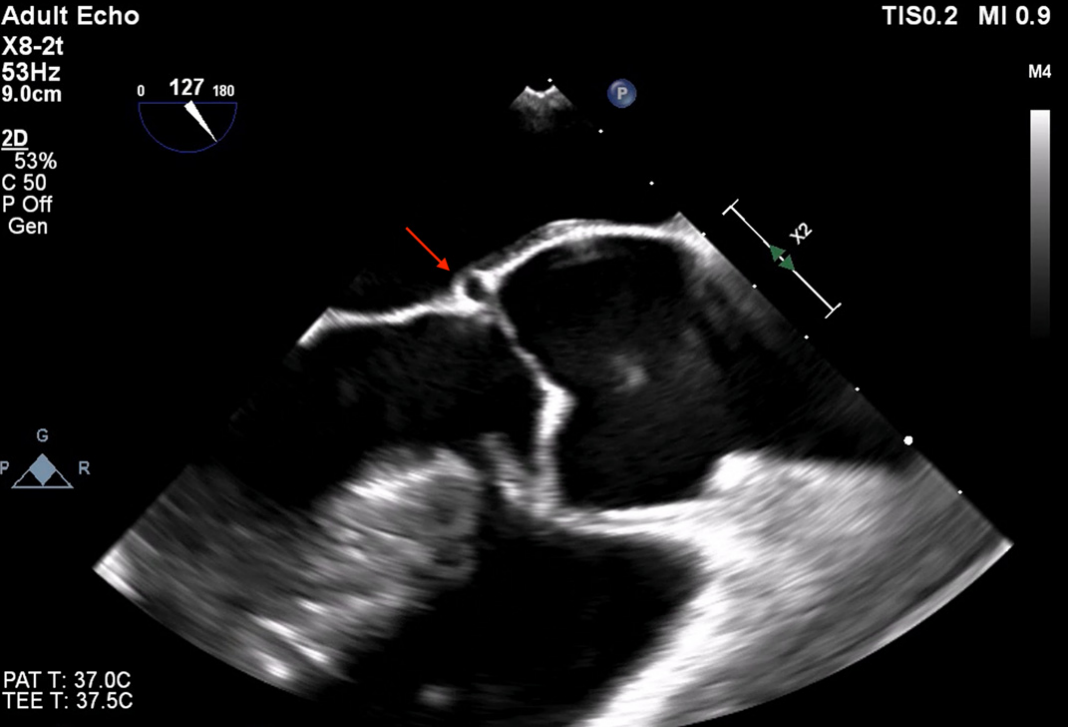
Midesophageal Long-Axis View The aberrant circumflex coronary artery at the level of the aortic annulus is visible (arrow). Echo = echocardiogram; other abbreviations as in Figure 1.
